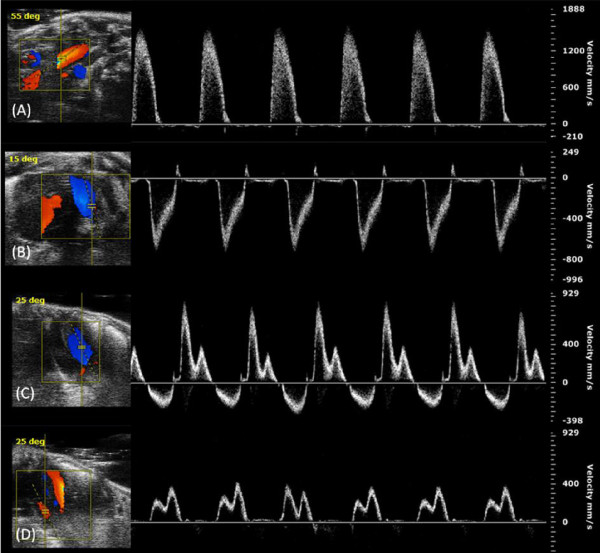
Representative Doppler echocardiographic images. (A) Suprasternal view (left panel) and PW Doppler tracing (right panel) of aortic outflow. (B) Modified short axis view (left panel) and PW-Doppler tracing (right panel) of pulmonary outflow. (C) Apical 4 chamber view (left panel) and PW-Doppler tracing (right panel) of mitral valve (MV) inflow. (D) Apical 4 chamber view (left panel) and PW-Doppler tracing (right panel) of tricuspide valve (TV) inflow.
